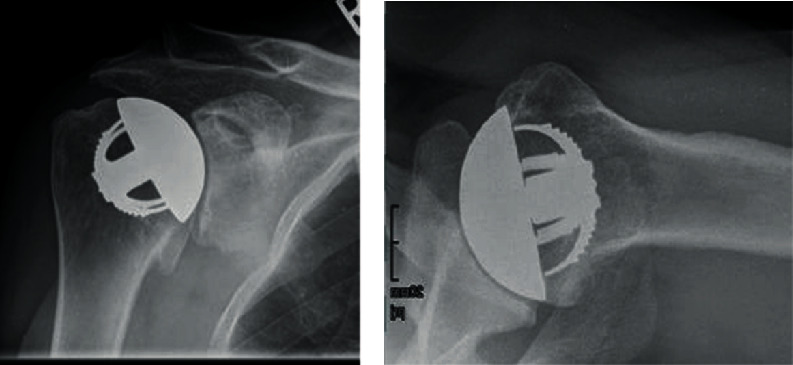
Preoperative X-ray, indwelling TESS anatomical stemless implant.
